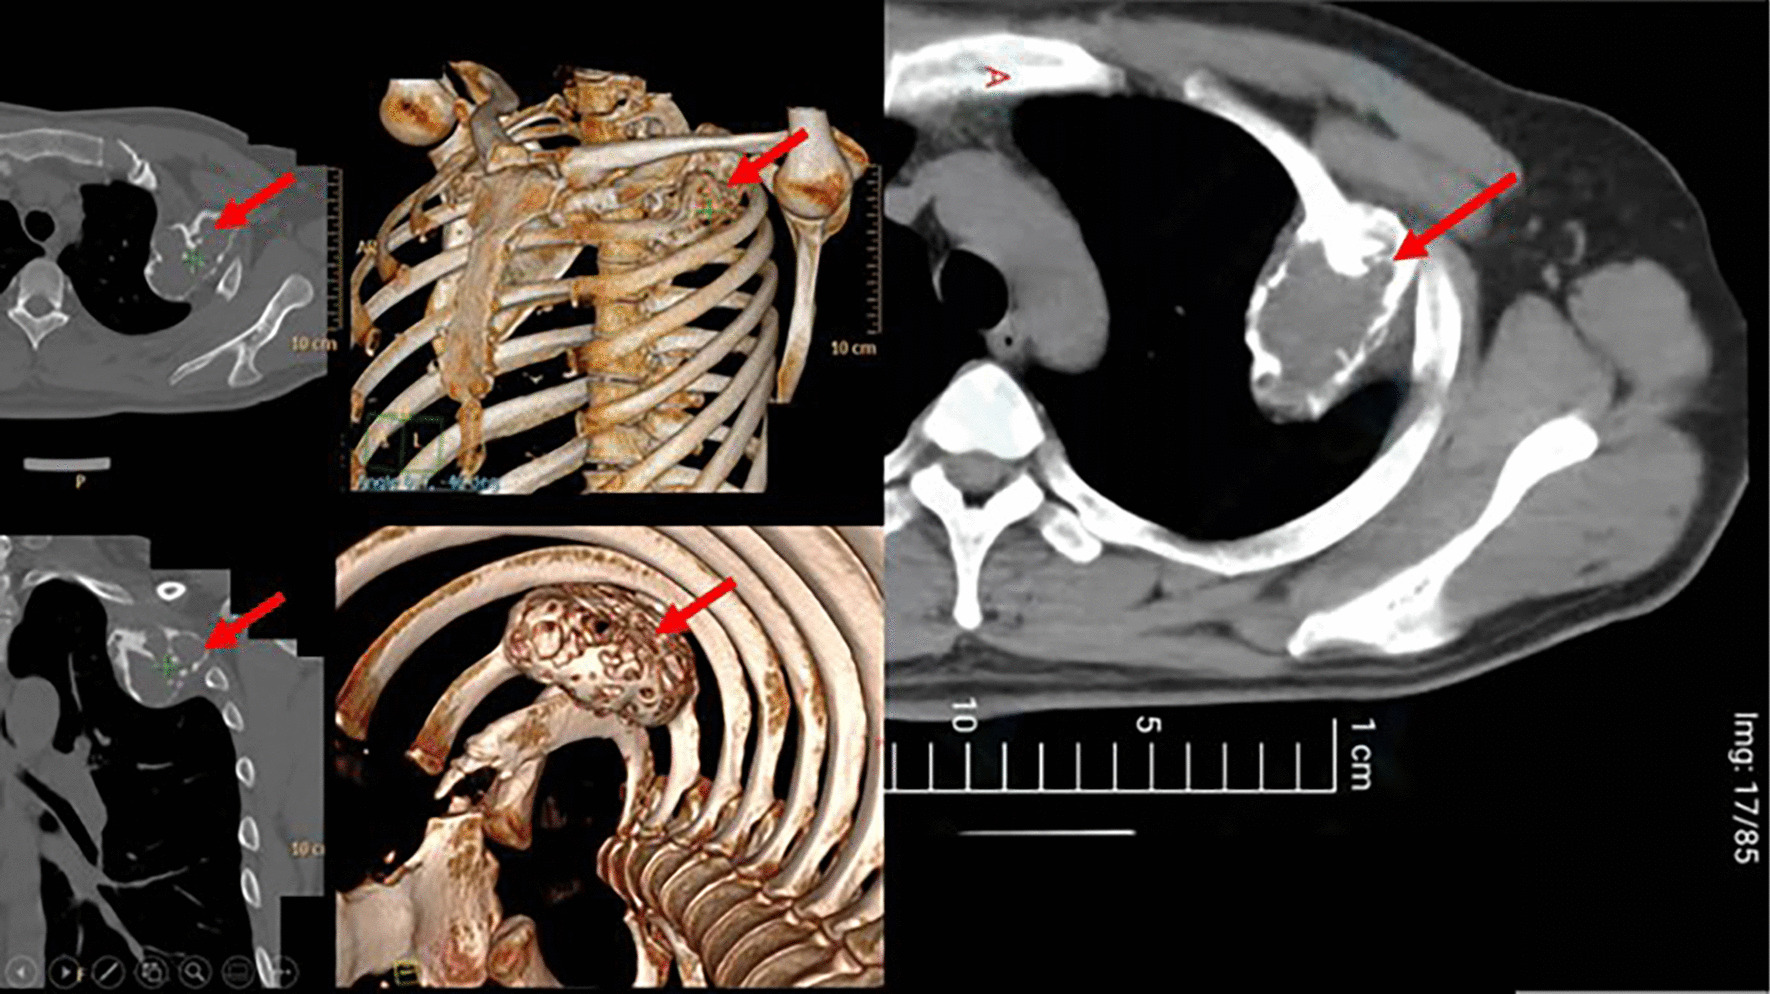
Chest CT and 3D reconstruction of fusiform lesion. The 3D reconstruction shows a mass in the left second rib (red arrow)
